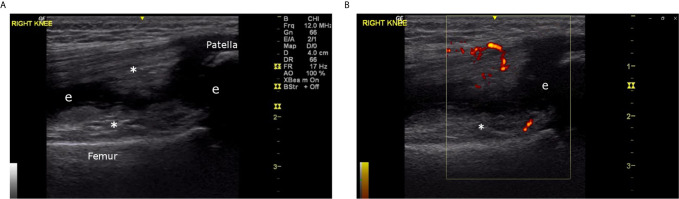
(A) Right knee, superior longitudinal view, greyscale (6 year old male, Case 2), demonstrating abundant proliferative synovitis (*) and effusion (e). (B) Right knee, superior longitudinal view, color Doppler (6 year old male, Case 2), demonstrating proliferative synovitis with intense 3+ Doppler signal (D).
